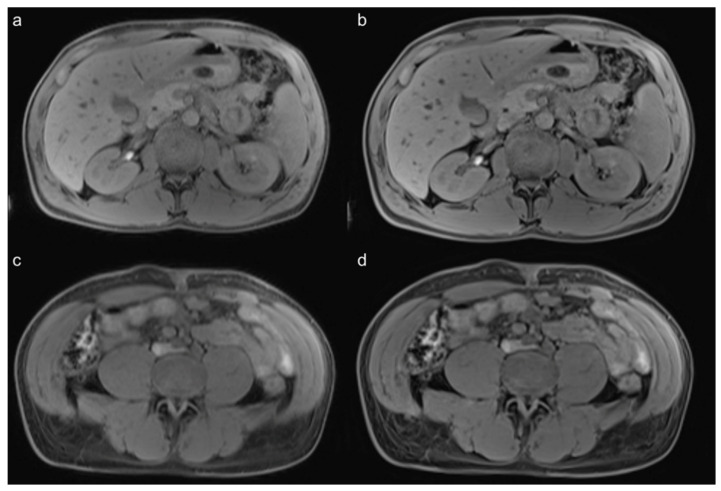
Precontrast images of a 28-year-old male patient with unclear elevation of the AFP-levels in his blood samples. In the MR images which were made to exclude an extragonadal tumor, the noise, organ sharpness, and sharpness of the intestine are better in the VIBE_SR_ (b,d) than in the VIBE_Std_ (a,c).
